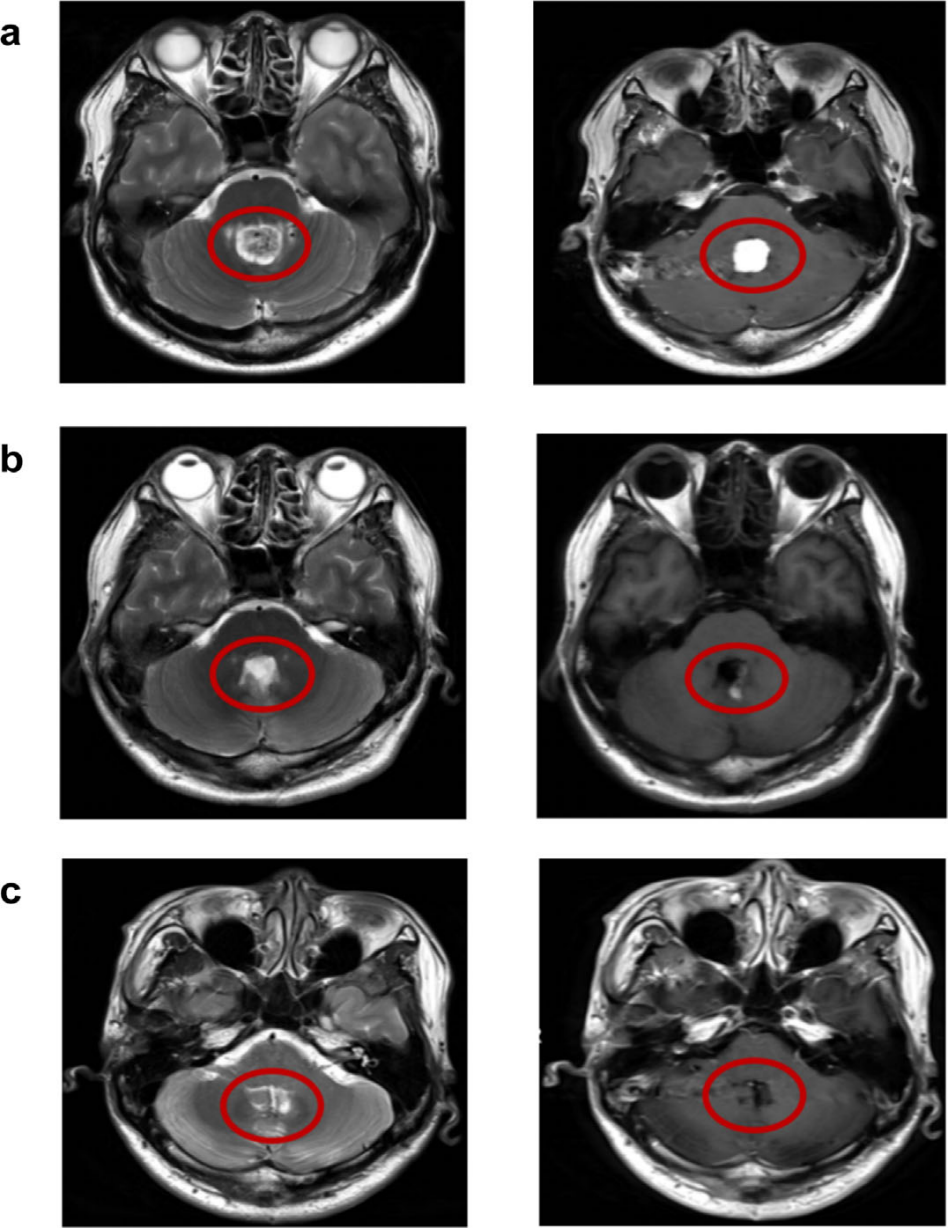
Pre- and postoperative brain magnetic resonance imaging scans of the patient. (a) Preoperative hemangioblastoma in the fourth ventricle/vermis. (b) One week after resection, marked edema. (c) Three months after resection. In each panel, the left image is T2-weighted, and the right image is T1-weighted. The red circle indicates the hypointense signal in the midline cerebellar vermis.
